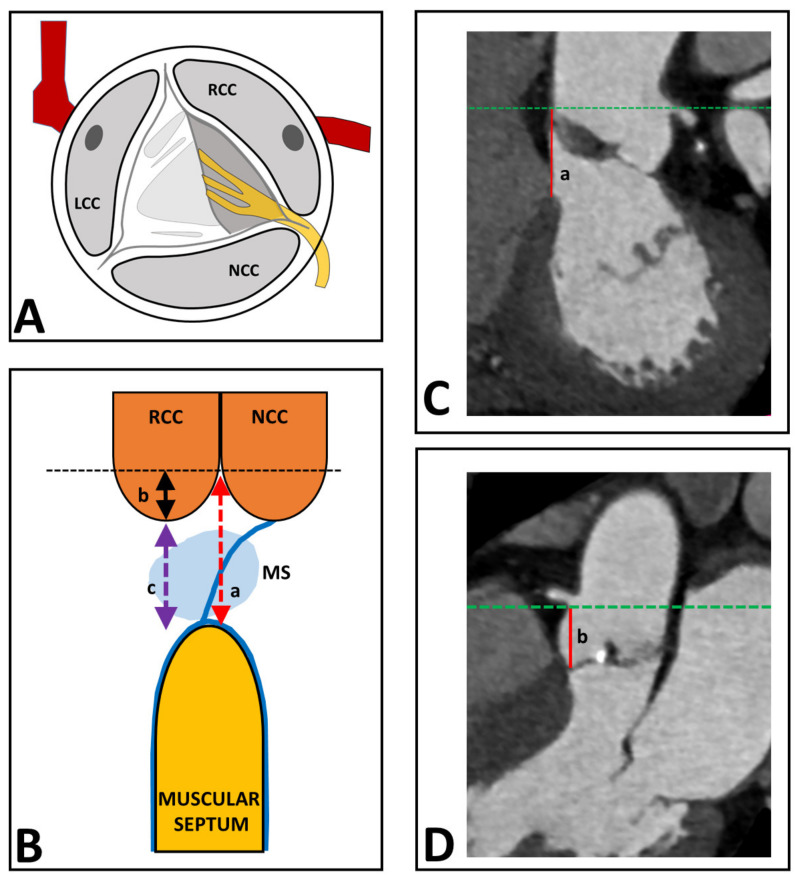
CT analysis of determination of EDACS from the CT scan. (A) shows the His-bundle that runs from the aortic valve (between the NCC and RCC) in an oblique trajectory towards the muscular septum. (B) shows the distance between the sinotubular junction and the muscular septum (line a), the depth of the RCC, measured from the sinotubular junction to the deepest point of the RCC (line b), and EDACS, which is calculated as the difference between line a and b (line c). (C) shows the distance between the sinotubular junction and the muscular septum (line a) measured on the CT scan. (D) shows the depth of the RCC, measured from the sinotubular junction to the deepest point of the RCC (line b) on the CT scan. EDACS, effective distance between aortic valve and conduction system; LCC, left coronary cusp; MS, membranous septum, NCC, non-coronary cusp; RCC, right coronary cusp.
